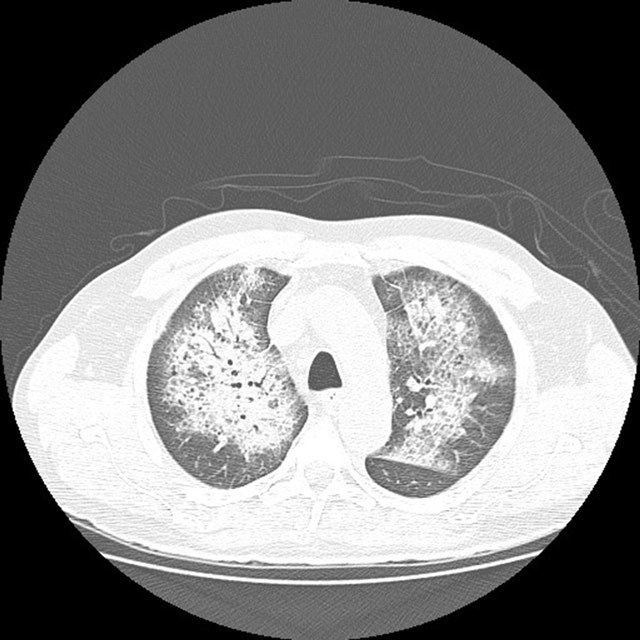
High resolution chest CT reveals interlobular septal thickening and ground-glass opacity in the upper perihilar areas, the so-called “crazy-paving” pattern.
